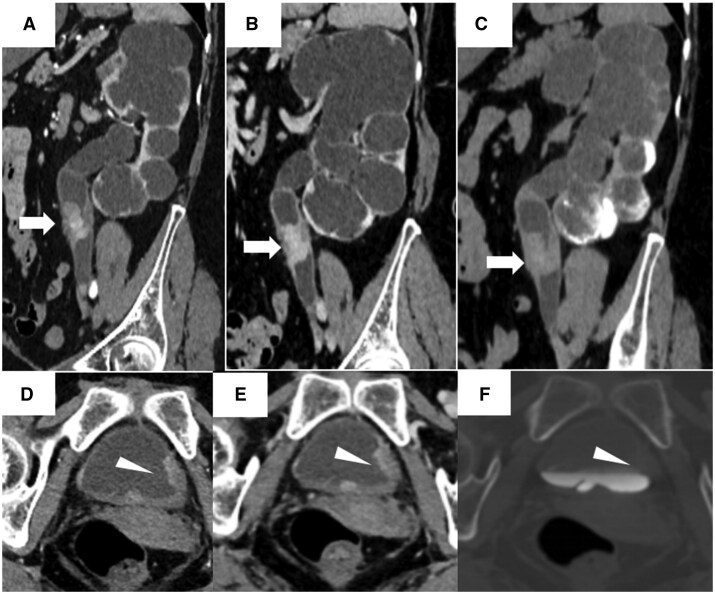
CT urography of ureteral and bladder malakoplakia. (A, D) Arterial phase; (B, E) venous phase; (C, F) excretory phase. (A-C) Axial images reveal annular thickening of the left upper ureter (arrow) with a polypoid, enhancing intraluminal mass, causing significant left-sided hydronephrosis. (D-F) Axial images demonstrate asymmetric thickening of the left posterior bladder wall (arrowhead) with marked mucosal enhancement.
